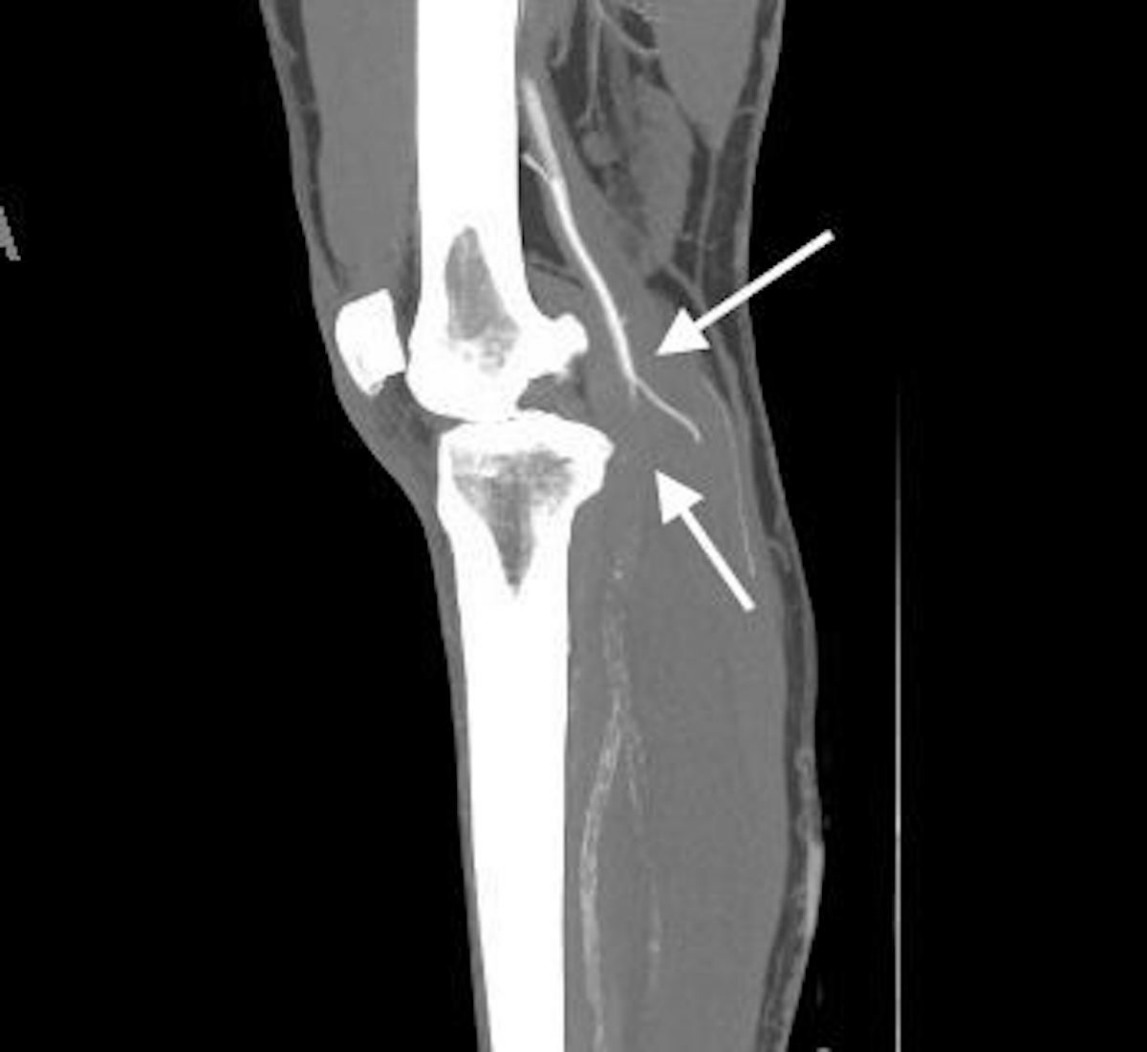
Computed tomography with contrast (sagittal plane) of left lower extremity demonstrating occlusion of the proximal popliteal artery.
